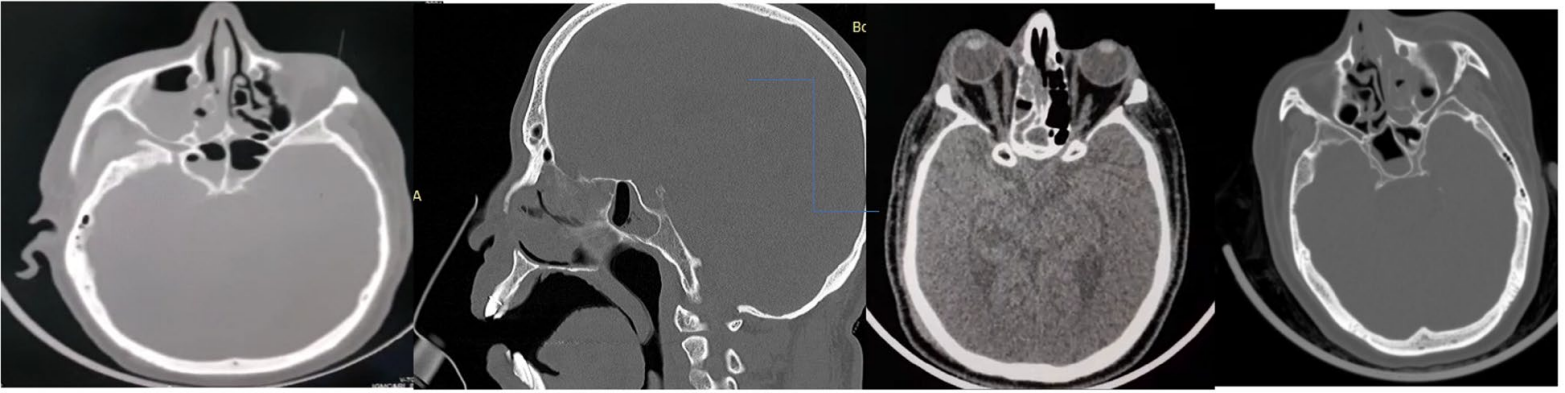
CT PNS of CAM patients showing involvement of maxillary, ethmoid and sphenoid sinuses, palatal necrosis, and skull base erosion
